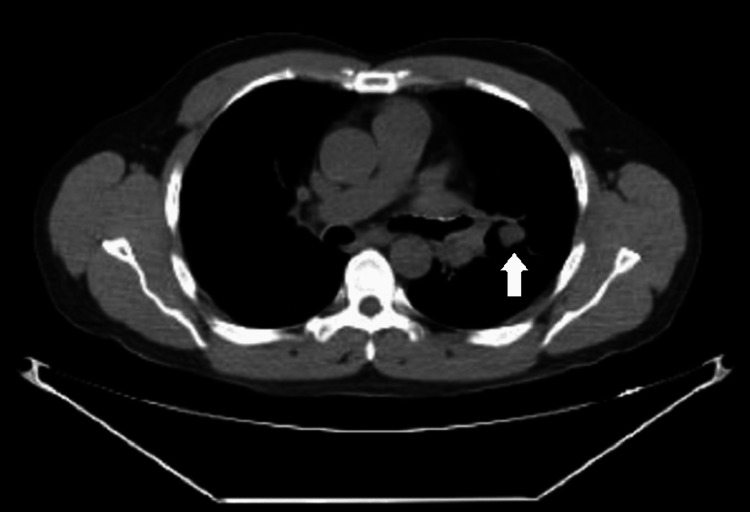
CT imaging of the left upper lobe. The left upper lobe nodule is visually indicated by a distinct white arrow.
